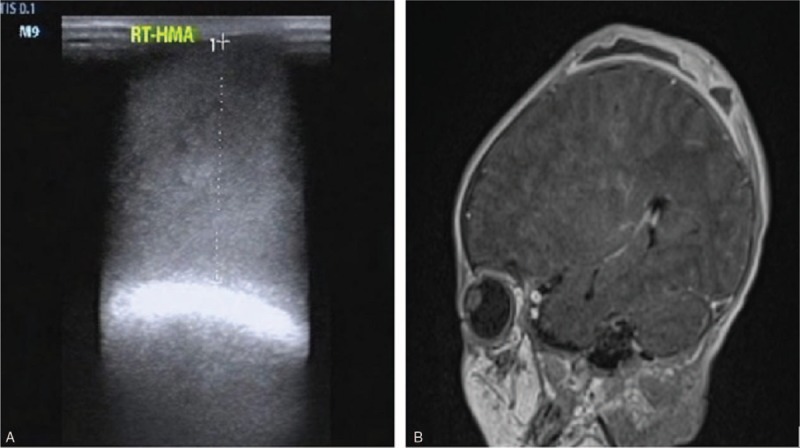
(A) B-mode ultrasound indicated the scalp mass was liquid and about 10.8 × 11.2 × 2.6 cm in size. (B) Enhanced MR examination, scalp had burst and no abnormalities were found in the medial side of the skull and skull.
